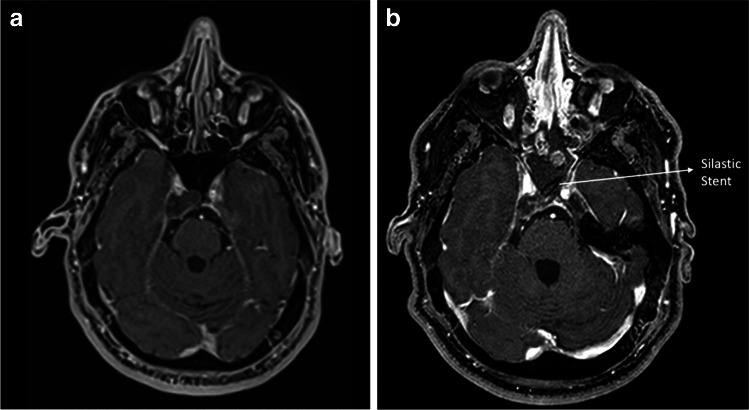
Preoperative (a) and postoperative (b) MRI of a patient undergoing endoscopic marsupialization of a biopsied right parasellar lesion
